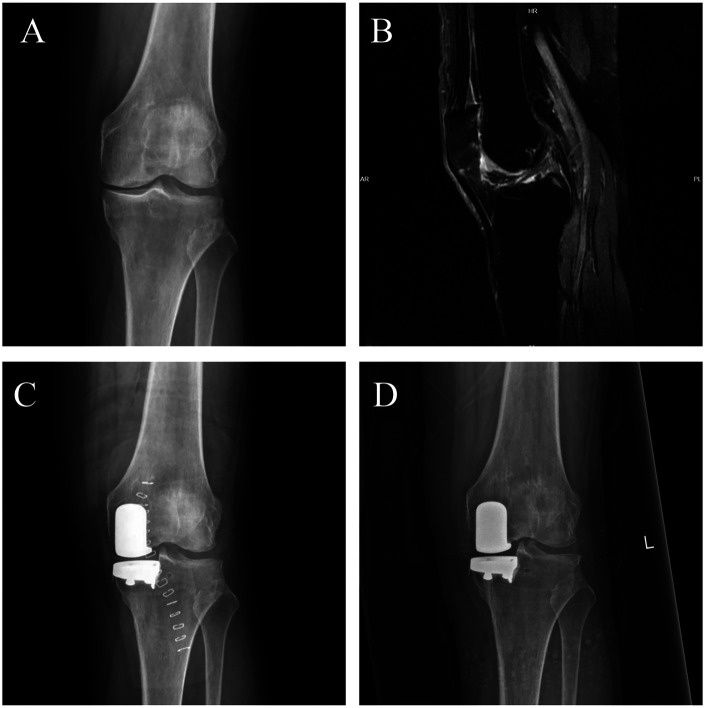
Representative case. (A) Preoperative radiograph showing medial joint space narrowing, subchondral sclerosis, and Kellgren–Lawrence grade III osteoarthritis. (B) Sagittal T2-weighted MRI demonstrating ACL elongation with partial fiber discontinuity, consistent with Grade II deficiency. (C) Postoperative radiograph at day 1 showing well-positioned fixed-bearing UKA components. (D) Five-year follow-up radiograph demonstrating maintained component alignment without loosening, subsidence, or lateral compartment progression.
